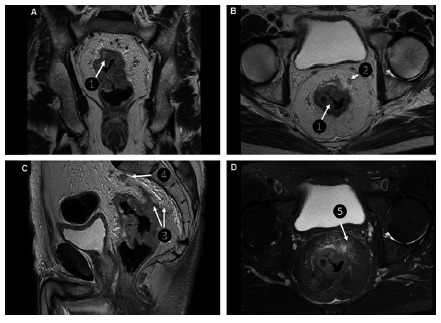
T2-weighted coronal (A) and axial (B) sequences demonstrating
